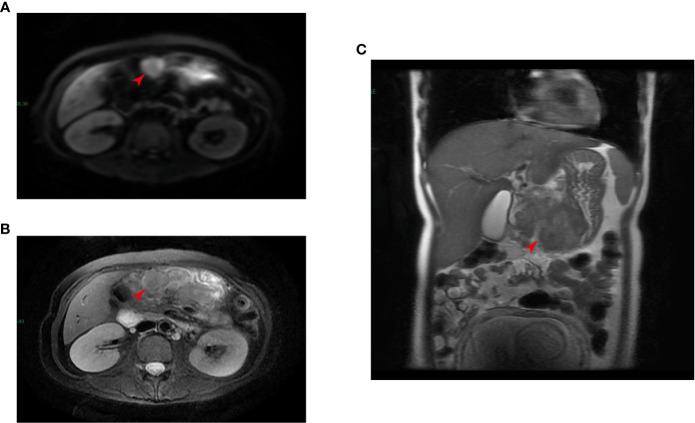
Magnetic resonance imaging (MRI) detected a tumor in the gastric sinus (as indicated by the red arrow, intersecting surface (A, B); coronal position (C)).
